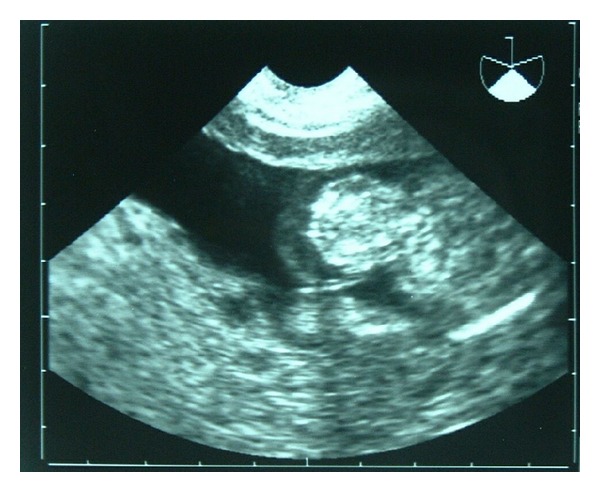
Ultrasound: fetus with omphalocele.
